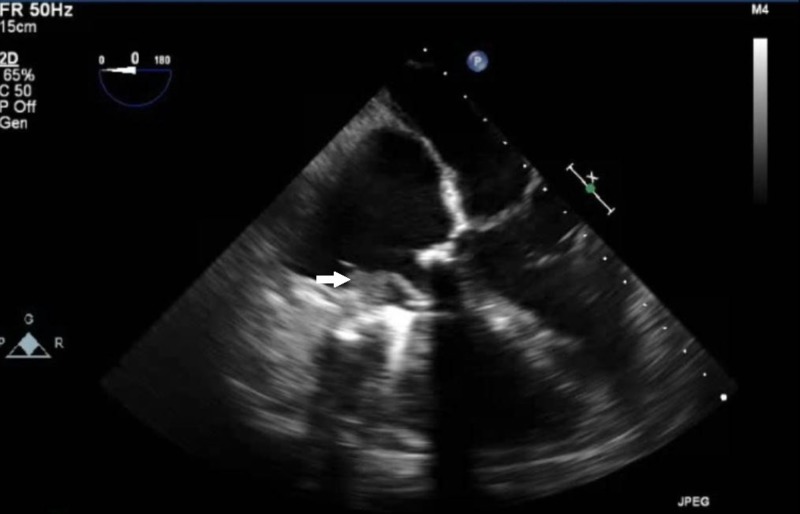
Transesophageal echocardiogram view of the tricuspid valve with evidence of a large thrombus (arrow) along the atrial aspect of the mechanical tricuspid valve
